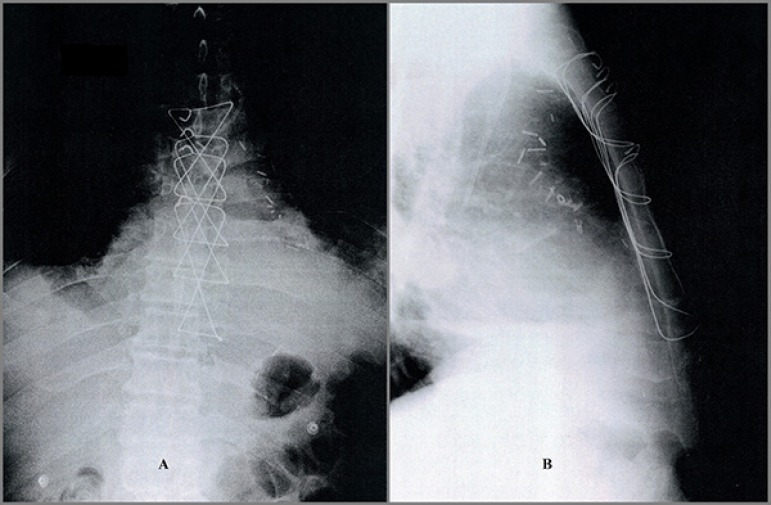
(A) Frontal and (B) lateral views on chest X-ray.
